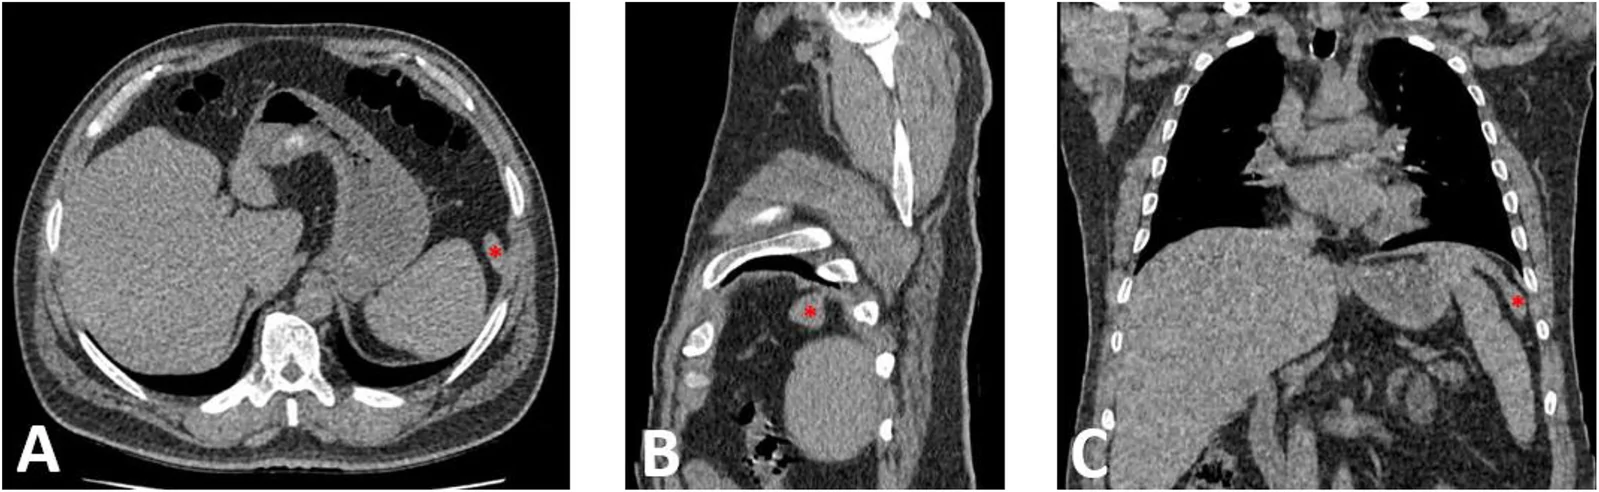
Noncontrast CT of the upper abdomen showing the elongated left hepatic lobe (beaver tail liver) mimicking a perisplenic mass. (A) Axial noncontrast CT image demonstrates the lateral tip of the elongated left hepatic lobe (red asterisk) wrapping around the spleen. The parenchymal attenuation of this extension is identical to the rest of the liver. (B) Sagittal and (C) coronal reformatted CT images clearly show the uninterrupted anatomical continuity of the tissue (red asterisks) with the left hepatic lobe, tracking superiorly and laterally along the splenic margin.
